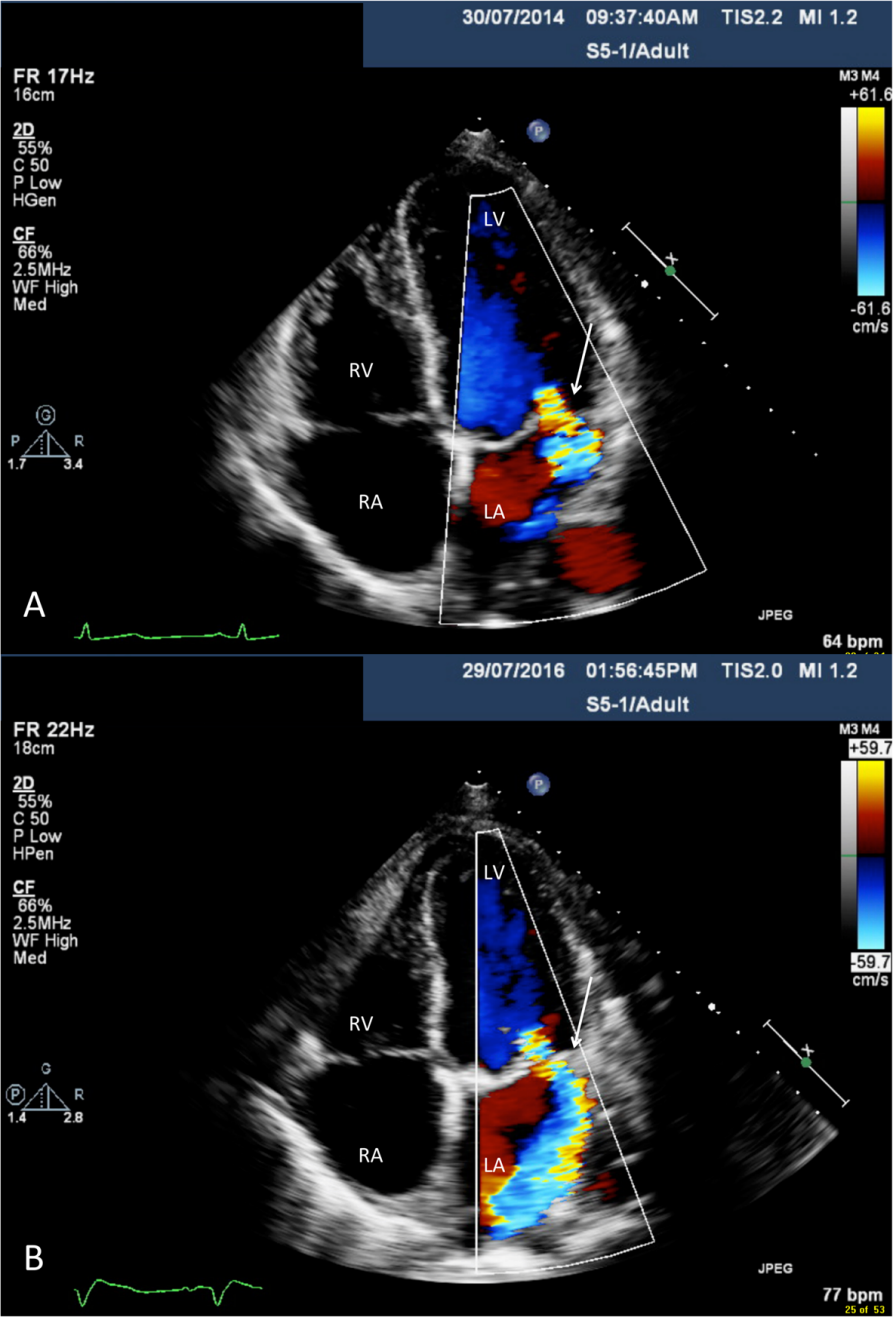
Apical four chamber view transthoracic echocardiogram findings in 2014 (a) and 2016 (b). Moderate-to-severe mitral regurgitation (MR) is noted with interval progression to severe MR in 2016
